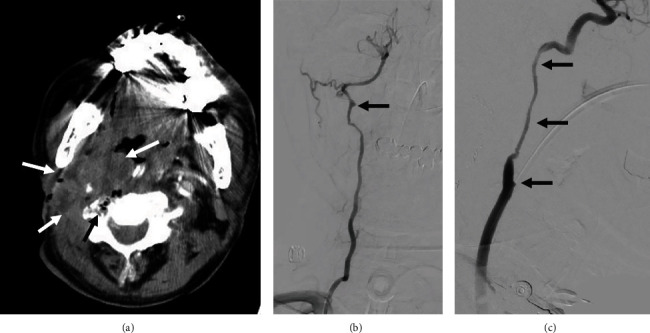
(a) Computed tomography angiography showed encasement of the right common carotid artery, right internal carotid artery, and right vertebral artery by the confluent hypoenhancing tumor (white arrows). Note the presence of air-containing necrosis around the right vertebral artery (black arrow). Angiography of the (b) right vertebral artery and (c) right common carotid artery showed focal vascular irregularity caused by tumor invasion (black arrows).
